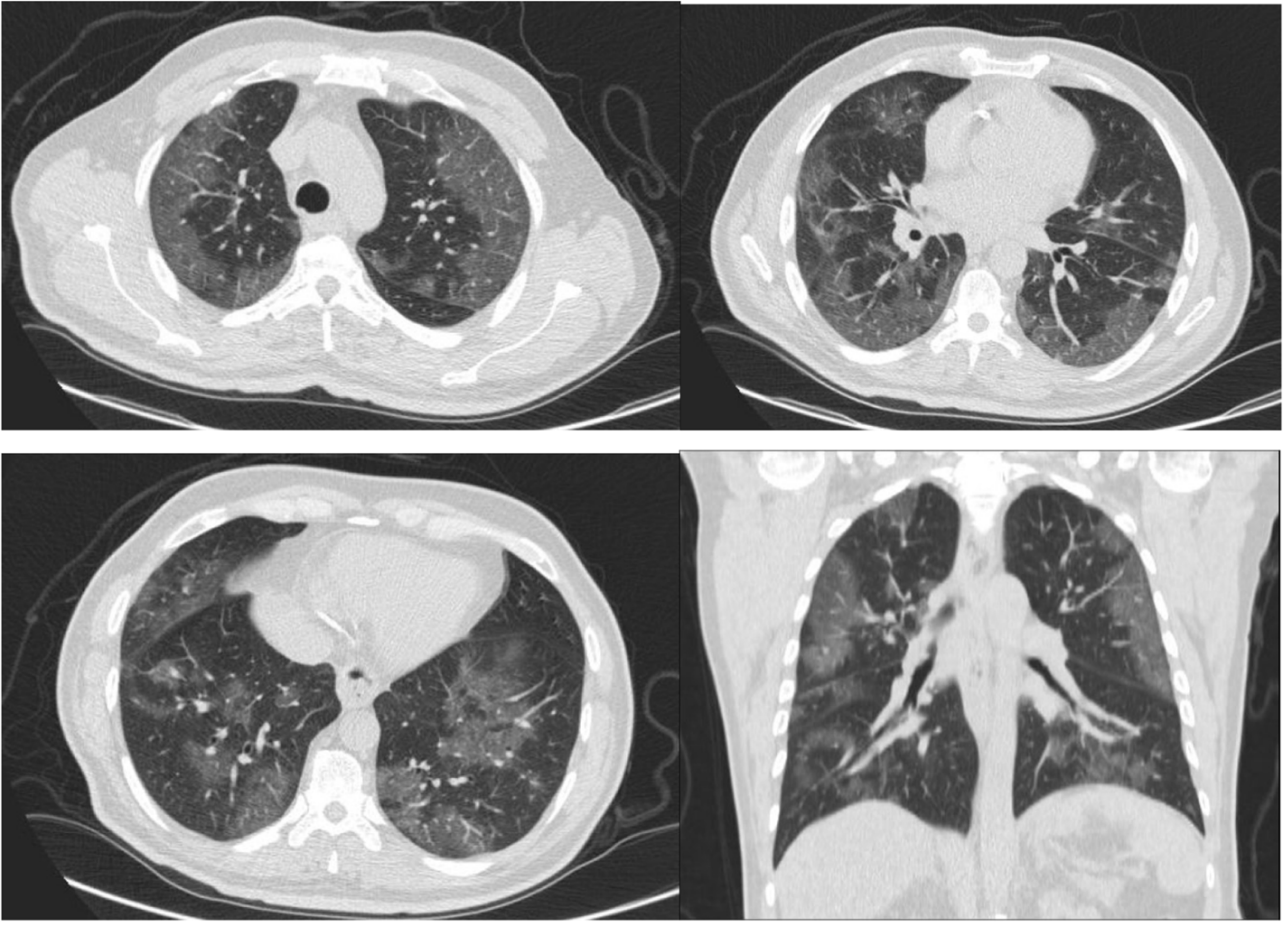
COVID-19 pneumonia: thin section CT shows bilateral multifocal subpleural and peribronchial GGO, semiqutitative score:18
